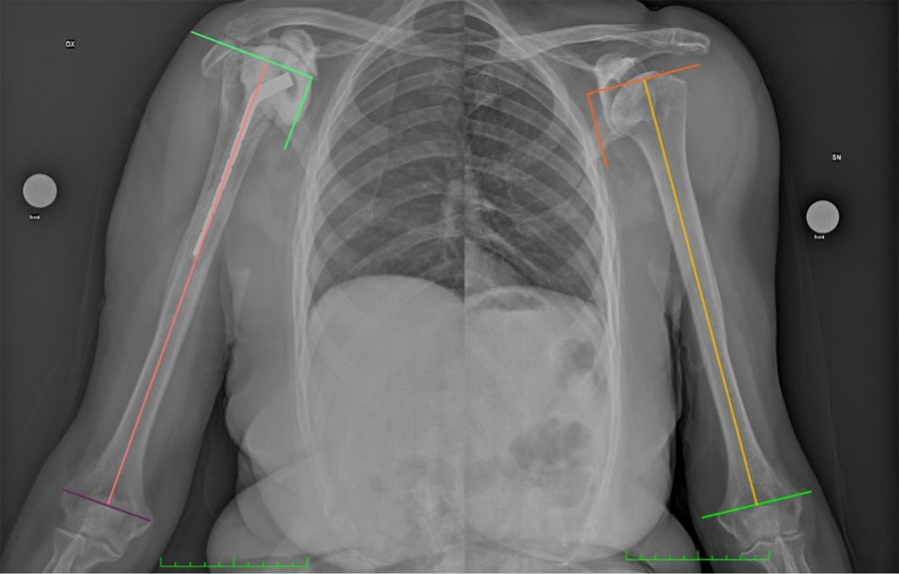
True anteroposterior bilateral scaled radiographs of humeri, captured with neutral rotation and the patient in a standing position. The humeri were laid flat against the cassette, and the X-ray beam was precisely directed at the middle third. To account for magnification, a centimeter marker was strategically positioned laterally to the humerus
